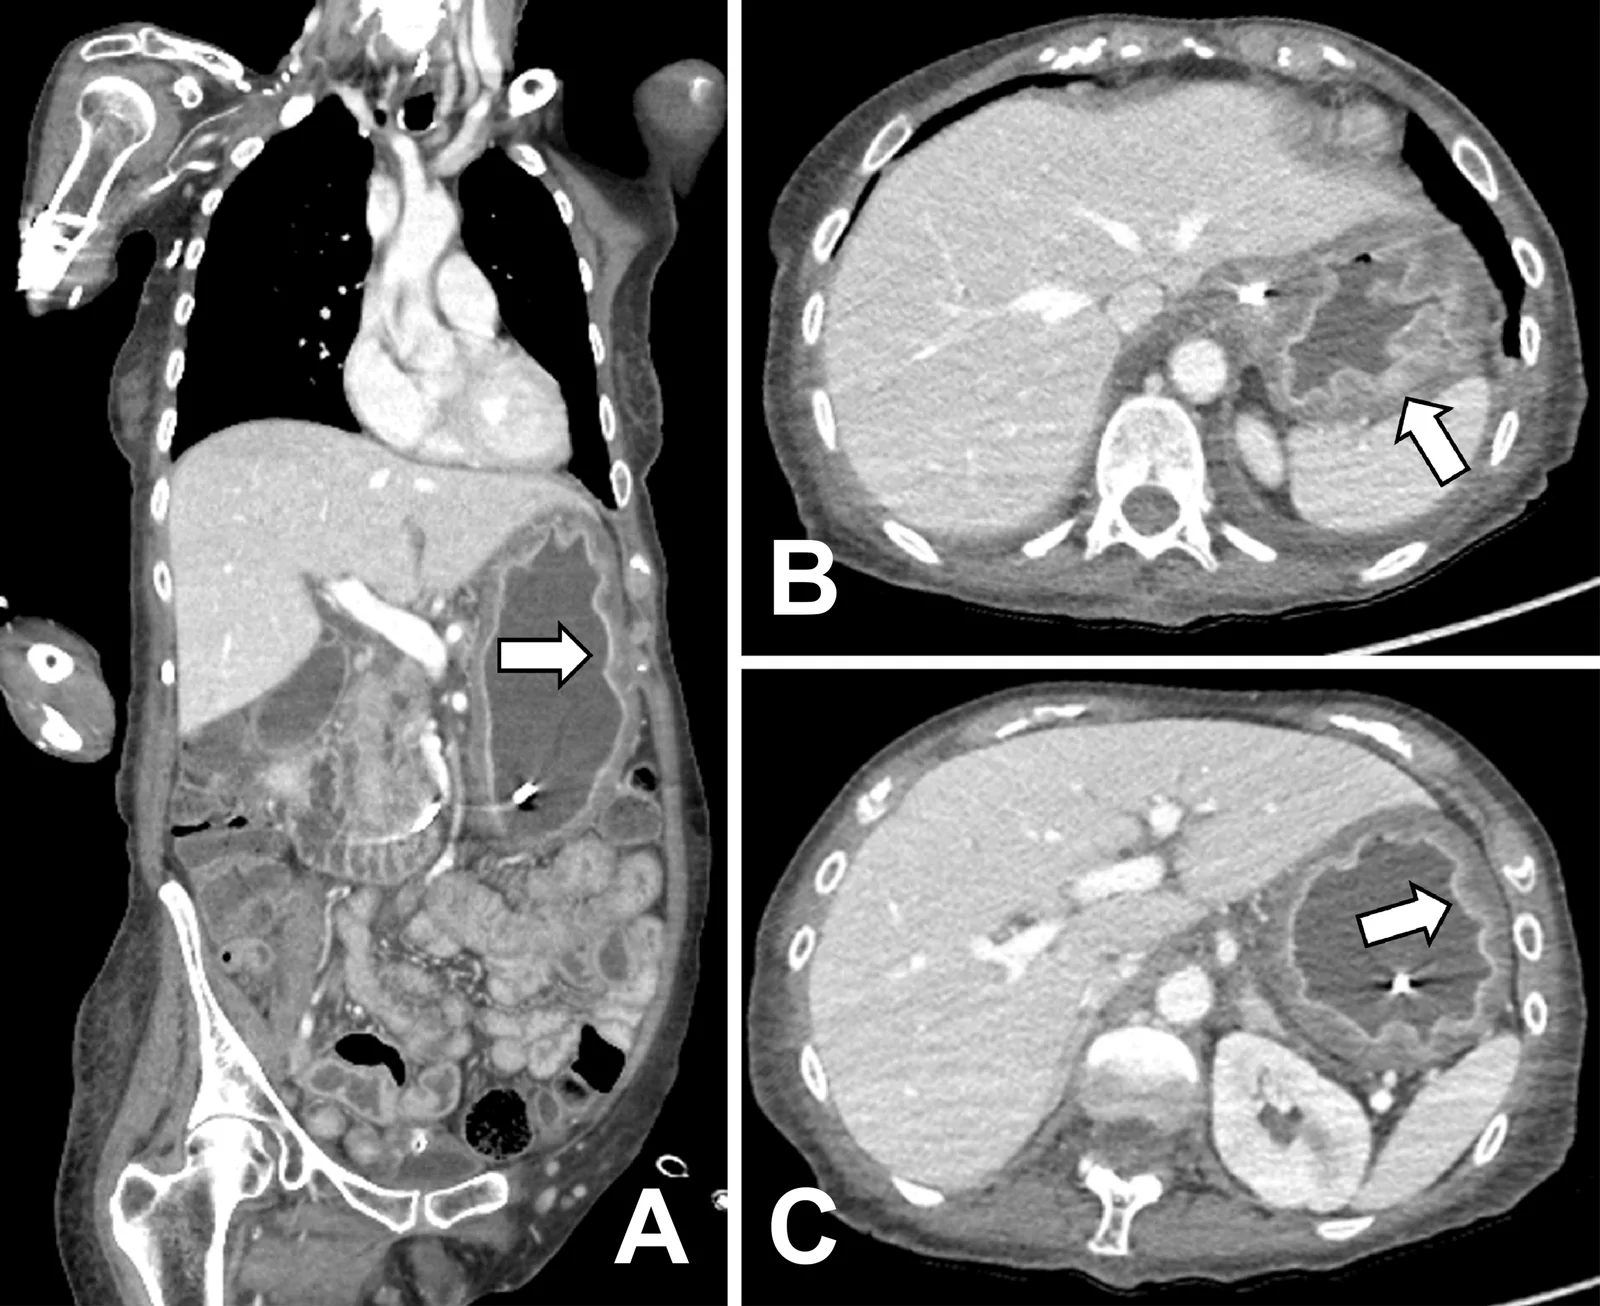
Radiological CT image of gastric amyloidosis (A) Coronal reformats of CT of the thorax, abdomen and pelvis and (B and C) axial reformats of the upper abdomen. A and C show a dilated stomach with diffuse gastric mural thickening (white arrows). Differential diagnoses include amyloidosis and linitis plastica, a malignant condition resulting from diffuse infiltration by a poorly differentiated carcinoma. Gastroscopy and biopsies from the mucosa and thickened gastric wall are critical for definitive diagnosis. CT: computed tomography This is an original image created by the authors.
